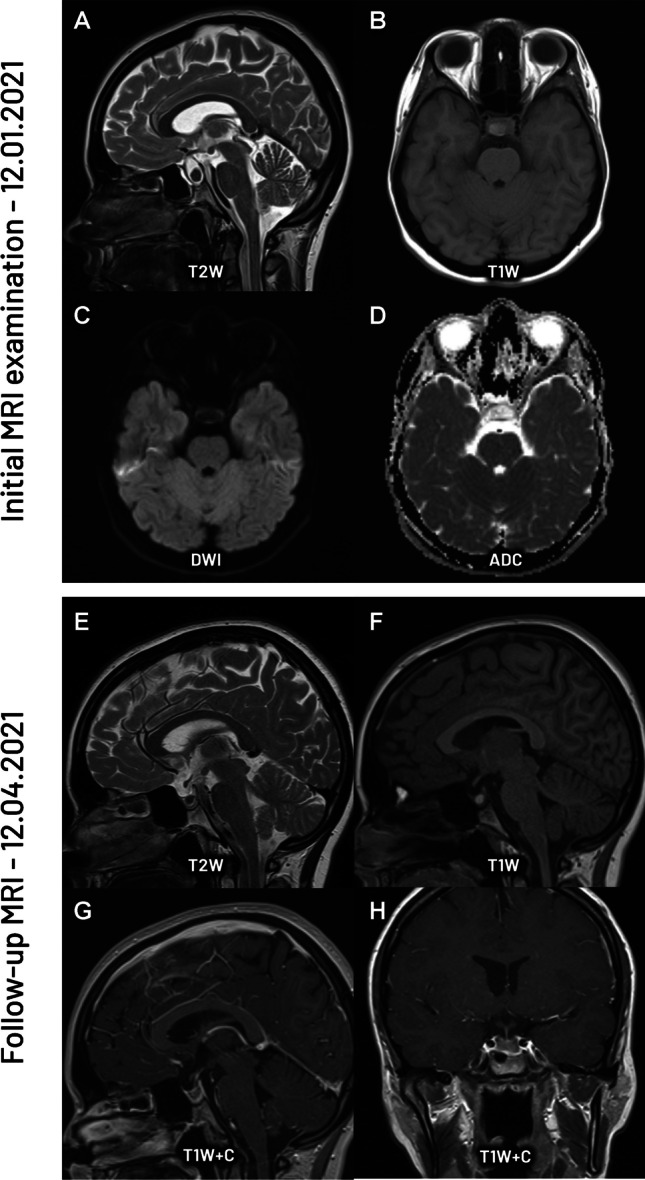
Initial MRI: sagittal T2-weighted image (A), axial T1-weighted image (B), axial DWI (C), ADC map (D). There is a tumor presenting high signal intensity region on T1 that was interpreted as a hemorrhage. On DWI (C), the tumor does not show features of reduced diffusion with high signal on the ADC map (D), indicating no bleeding but suggesting a high protein content. The follow-up MRI performed four months later confirms the initial diagnosis of Rathke’s cleft cyst, with regression of the size of the lesion on sagittal T2-weighted image (E), sagittal T1-weighted image (E), and post-contrast sagittal (G) and coronal (H) T1-weighted images
